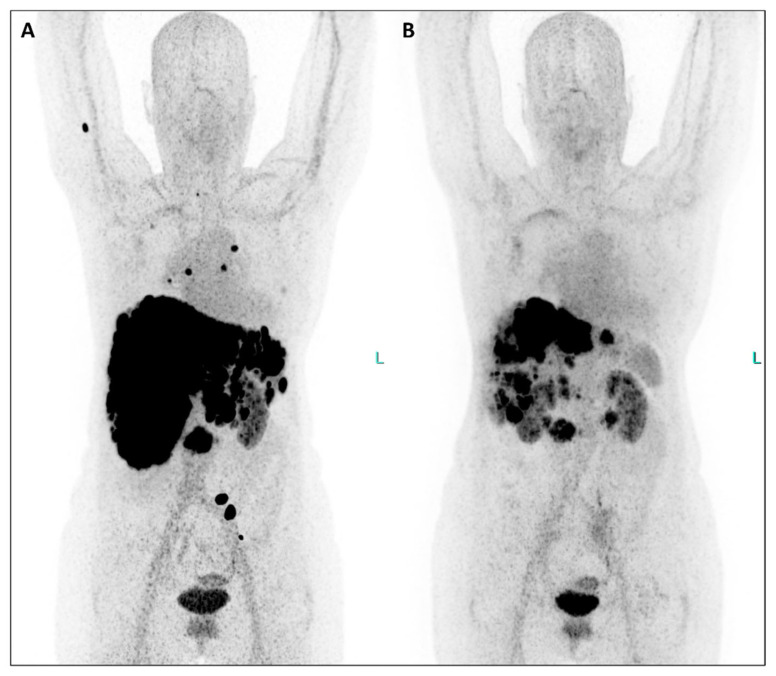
In July 2023, the patient underwent restaging PET/CT with the SSTR antagonist [^68^Ga]GaDOTA-LM3 ((A), maximum-intensity projection—MIP), revealing an extensive disease burden. Intense uptake of the SSTR antagonist was observed in the pancreatic head, multiple confluent foci in both liver lobes, presenting in a disseminated pattern alongside hepatomegaly and in mediastinal/hilar lymph nodes (Figure 1). Additionally, uptake was noted in several bone segments (right humerus, right scapula, some vertebral bodies, left ilium). Consequently, PRRT with the antagonistic peptide DOTA-LM3 (intravenously administered) was scheduled, involving both beta-emitting Lutetium-177 and alpha-emitting Actinium-225 as ^177^Lu/^225^AcDOTA-LM3 (TANDEM-PRRT). The patient underwent three courses of TANDEM-PRRT (July, September, and November 2023), with a total administered activity of 11.3 GBq for [^177^Lu]LuDOTA-LM3 and 26.4 MBq for [^225^Ac]AcDOTA-LM3. Therapy was well tolerated without significant acute adverse effects. MIP images from [^68^Ga]GaDOTA-LM3 PET/CT before the first (A) and second (B) course of TANDEM-PRRT are shown. Comparison of PET/CT after a single course of TANDEM therapy with the antagonist DOTA-LM3 showed an excellent response. Liver metastases and the primary pancreatic tumor exhibited extraordinary improvement, accompanied by a marked reduction in liver size. No significant DOTA-LM3 uptake was detected in the mediastinal/hilar lymph nodal metastases and most bone lesions, with only faint residual uptake in the left pelvis metastasis. Notably, no new distant metastases were detected.
